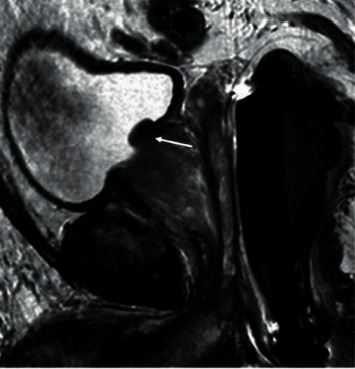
MRI of superselective intubation angiography showing hyperplasia (arrow).
